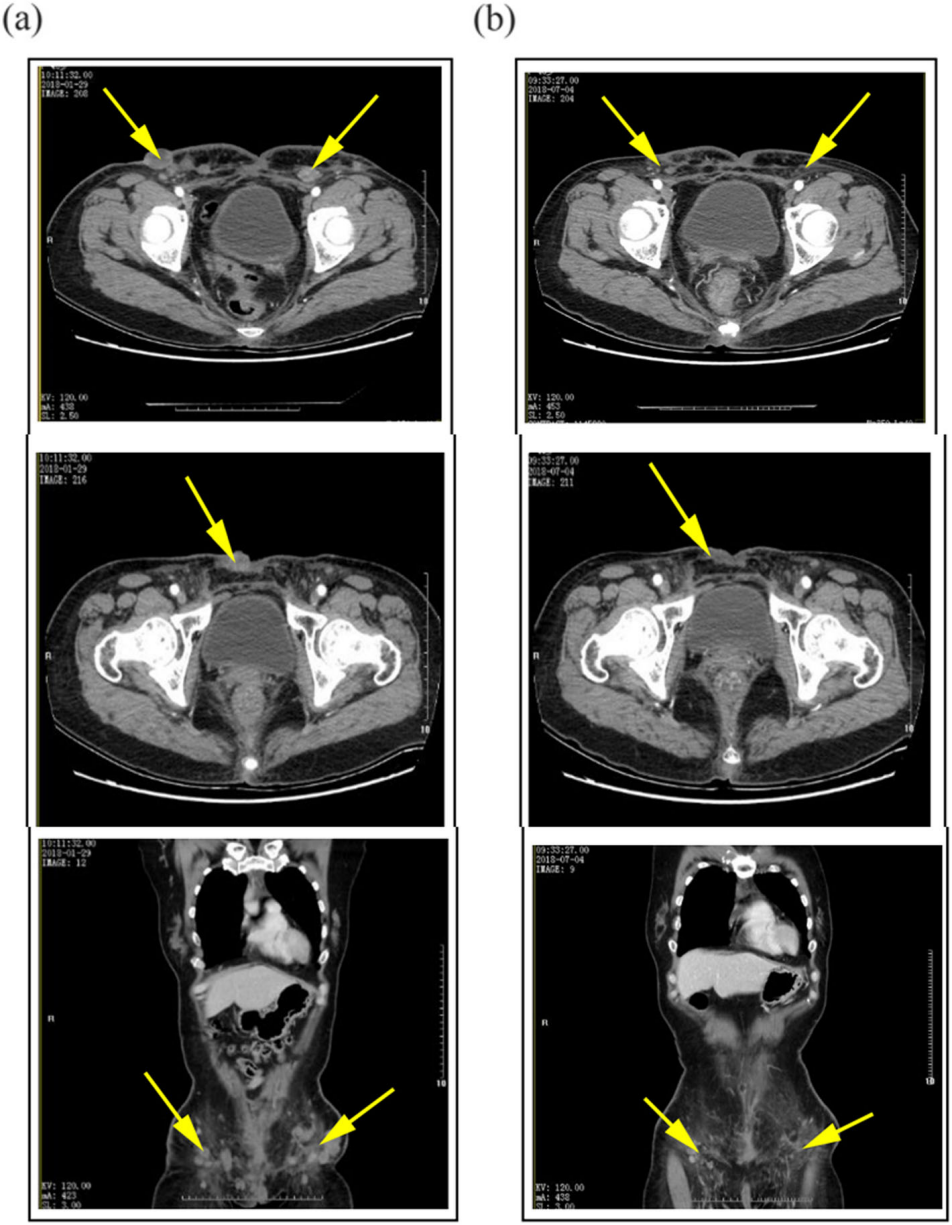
Contrasted CT scans showing the size and number of abdominal wall tumors decreased significantly after 6 months of oral apatinib treatment (axial and coronal). a Before treatment. b After 6 months of apatinib
